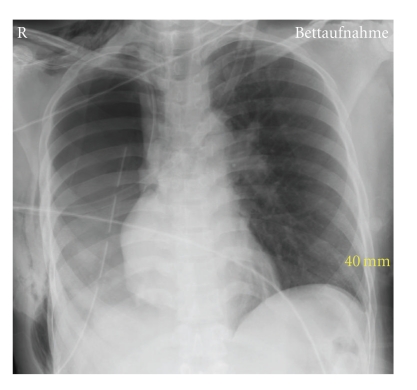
Chest X-rays of the patient immediately after pneumonectomy.
